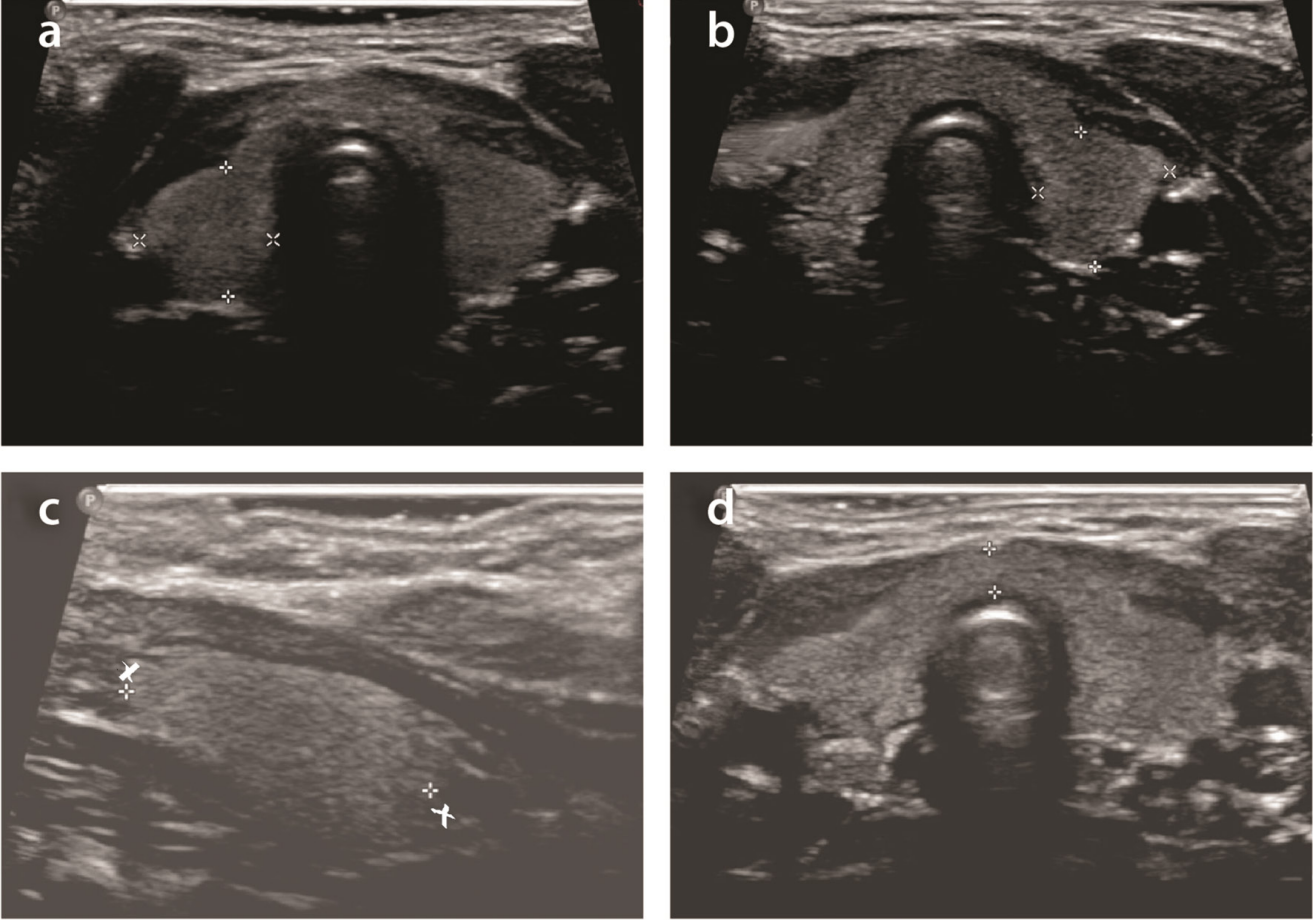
(a, b, c and d) Thyroid ultrasound images of normal infant showing width and anteroposterior measurement of right lobe (a), left lobe (b), length of right lobe (c) and anteroposterior measurement of the isthmus (d). Note that measurement of the thyroid isthmus is not factored into the formula for calculating thyroid volume.
